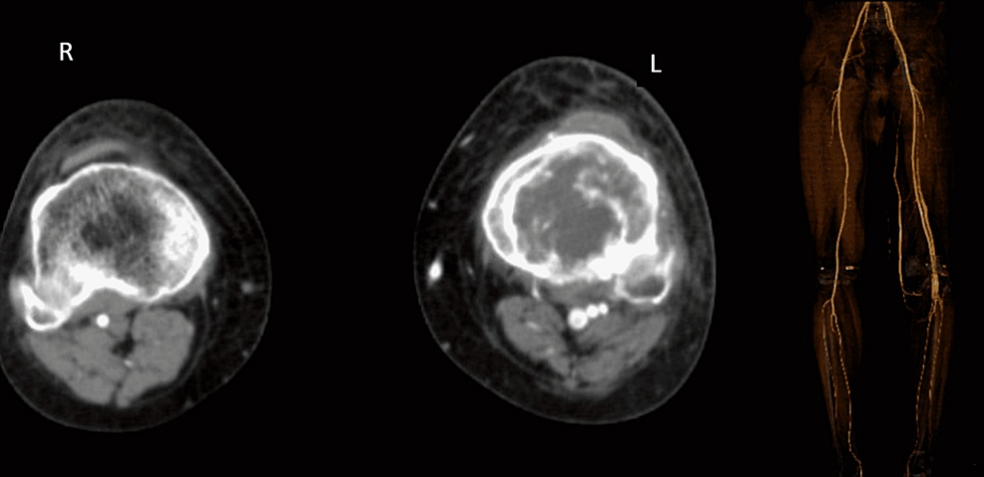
CT angiography suggestive of a tumor extending up to the anterior aspect of the popliteal vessel with no involvement of the vessels
